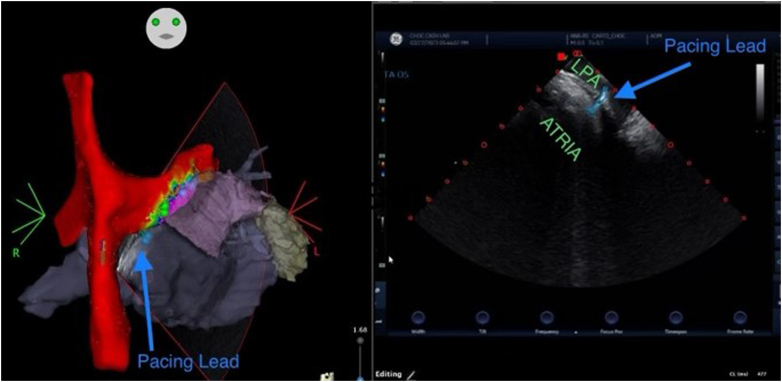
Integrated 3-Dimensional Image of Final Lead Placement (Left Panel) Multimodal three-dimensional image combining electroanatomical voltage map, computed tomography angiogram, and intracardiac echocardiography catheter location and ultrasound sector projected onto the map, with pacing lead outlined in light blue. Voltage map with color gradient: red = voltage <0.1 mV; purple = voltage >0.5 mV. (Right Panel) Intracardiac echocardiography image of pacing lead transversing the left pulmonary artery and affixed to the epicardial common atrium. LPA = left pulmonary artery.
